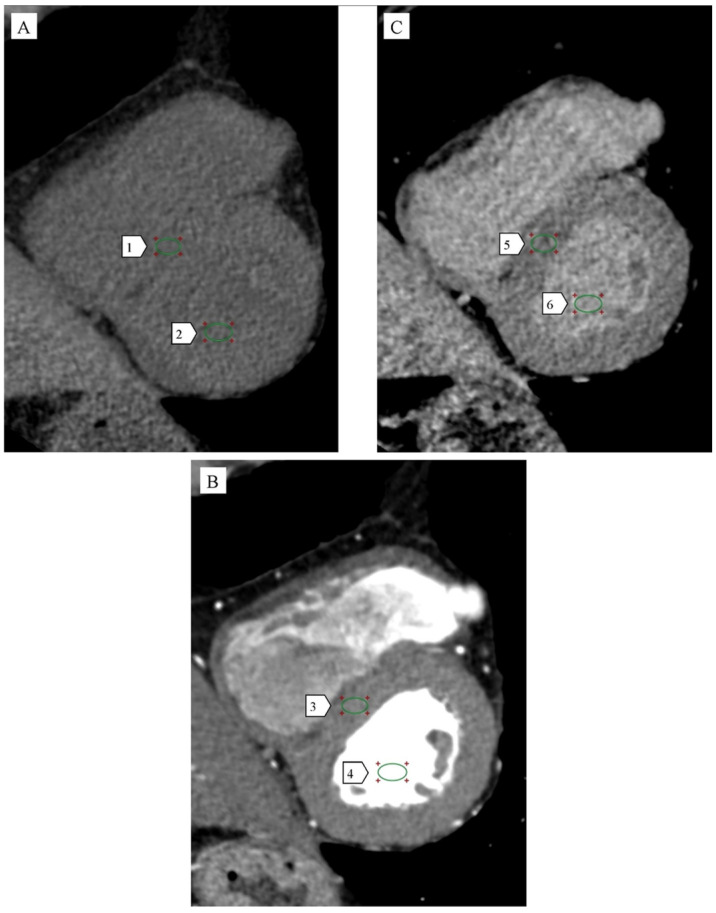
Cardiac computed tomography (CT). Attenuation method. Multiplanar reconstruction (MPR). Short axis. Level of middle segments of the left ventricle. (A) Native phase. 1. HU myocardium in the native phase: 43.83 HU. 2. HU LV blood in the native phase: 47.32 HU. (B) Angiographic phase CT (CCTA). 3. HU myocardium in CCTA: 112.31 HU. 4. HU LV blood in CCTA: 594.81 HU. (C) Delayed phase (CT-LE). 5. HU of myocardium in CT-LE: 78.07 HU. 6. HU LV blood in CT-LE: 111.73 HU. Hematocrit: 0.46. ECV: 28.7%. This figure represents original material created by the authors.
